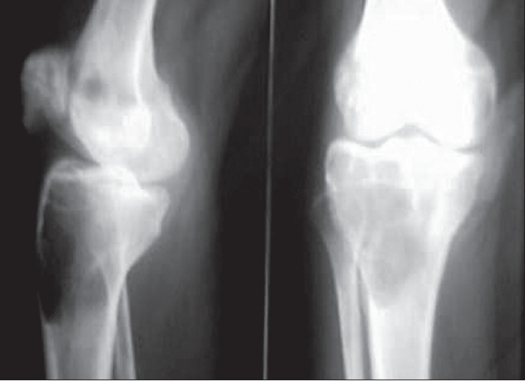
Pre Operative X-ray lateral and A.P. view shows Eccentric lytic lesions in upper end tibia, lower end femur & patella
